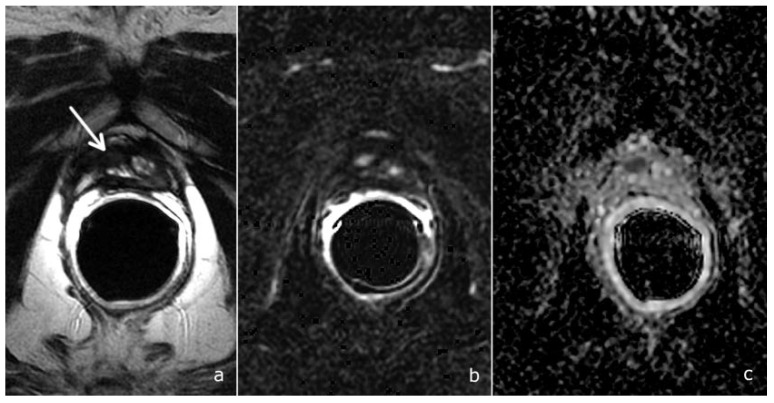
A PI-RR-score-5 patient, 70 years old, with a PSA value of 0.31 ng/mL. The patient underwent radical prostatectomy for bilateral prostate cancer, Gleason score 4 + 3, with bilateral surgical positive margins at the prostate apex. mpMRI documented a small hypointense tissue in the T2-weighted image, right-sided to the vesico-urethral anastomosis (a-arrow), characterized by intense and early contrast enhancement (b) and restricted diffusion at the ADC map, (c) PI-RR score = 5.
